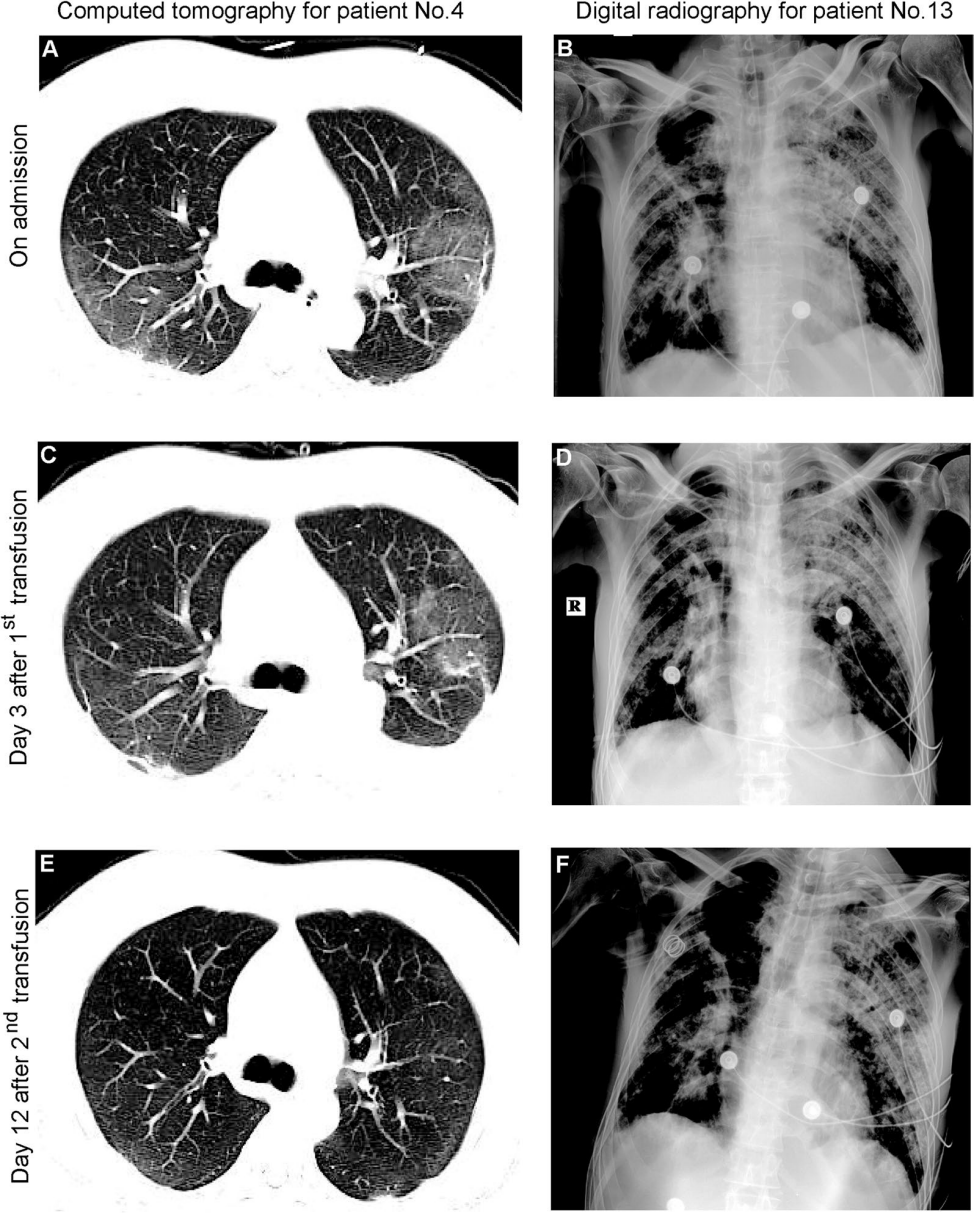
Dynamic changes to chest radiologic images after CP transfusion. (A) Ground-glass opacity was observed in bilateral lobules by computed tomography images for patient No.4 on admission to the intensive care unit. (B) Patchy shadows of high density were detected in digital radiography for patient No.13 on admission. (C) On day 3 after 1^st^ convalescent plasma transfusion, alleviation of lesions was noticed for patient No.4. (D) No substantial improvement was detected for patient No.13. (E) On day 12 after 2^nd^ convalescent plasma transfusion, notable alleviation was detected for patient No.4. (F) No substantial improvement was detected for patient No.13
